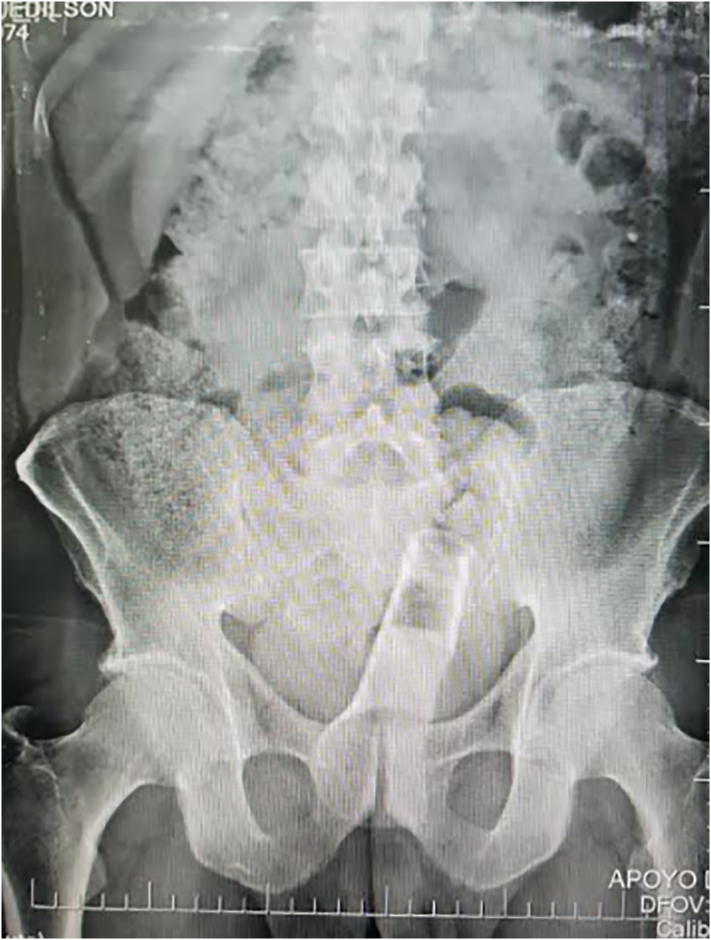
X-ray showing radiopaque image compatible with foreign body in rectum.
